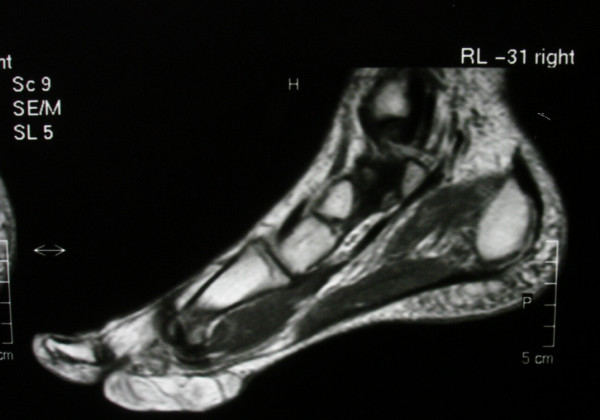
The sagittal T1-weighted image. A lobulated, encapsulated, fatty mass with multiple hypointense nodules and septa in the subcutaneous layer of the forefoot, underneath the plantar aponeurosis.
